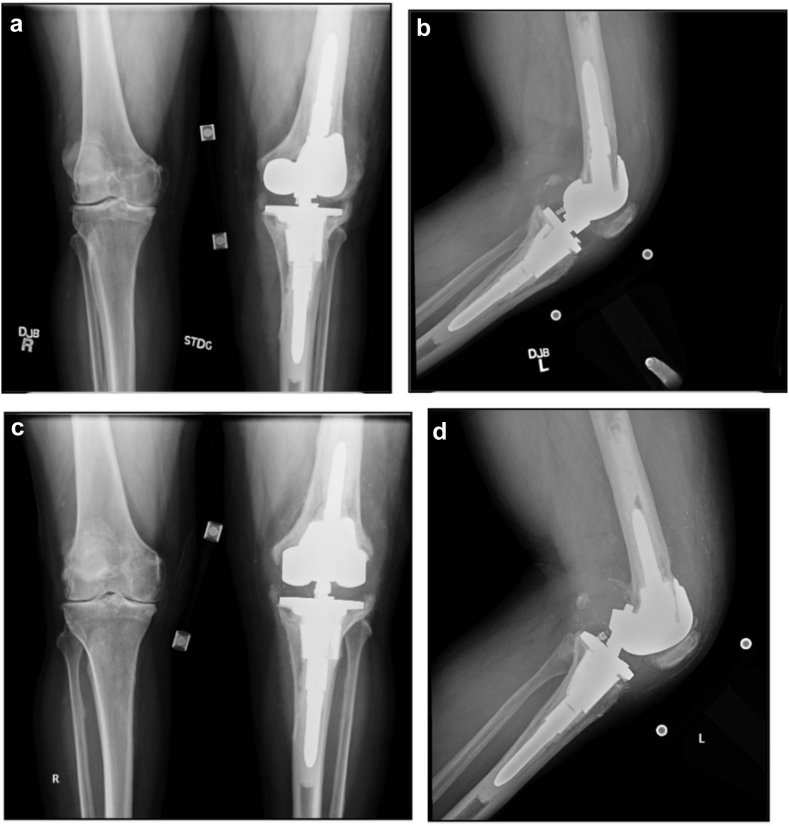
(a) Anteroposterior and (b) lateral radiographs of the single mechanical failure case secondary to aseptic loosening of the femoral component before the first revision. (c) Anteroposterior and (d) lateral radiographs of the previously revised RHK for mechanical failure before the second revision surgery which was indicated for a bent hinge mechanism.
